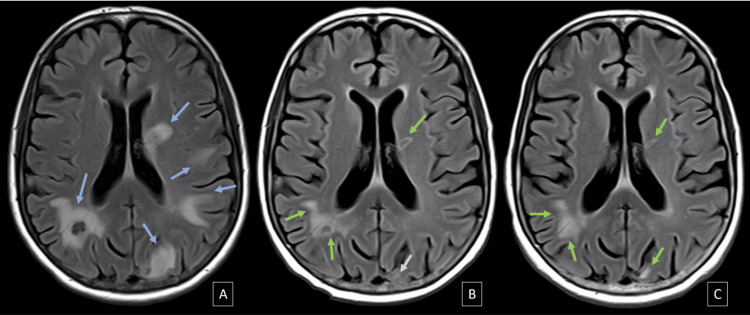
Axial FLAIR of a 64-year-old female patient with metastatic lesions from undifferentiated large cell lung cancer FLAIR: Fluid-attenuated inversion recovery; MRI: magnetic resonance imaging; SRS: stereotactic radiosurgery In the baseline MRI (A), prior to SRS, multiple areas of pronounced perilesional edema surrounding the metastatic lesions are shown (blue arrows). In MRI-2 (B) at three months and MRI-3 (C) at six months, there is a progressive decrease in the distribution and extent of perilesional edema, associated with a reduction in lesion size (green arrows)
